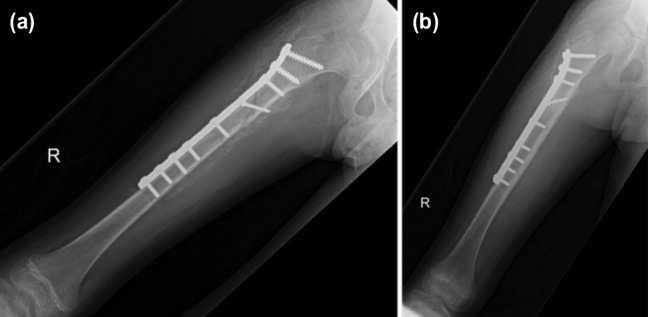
(a) The radiograph showing the involucrum in the proximal half of the femur. (b) The radiograph showing union of the fracture and resolution of infection 10 months after injury.
